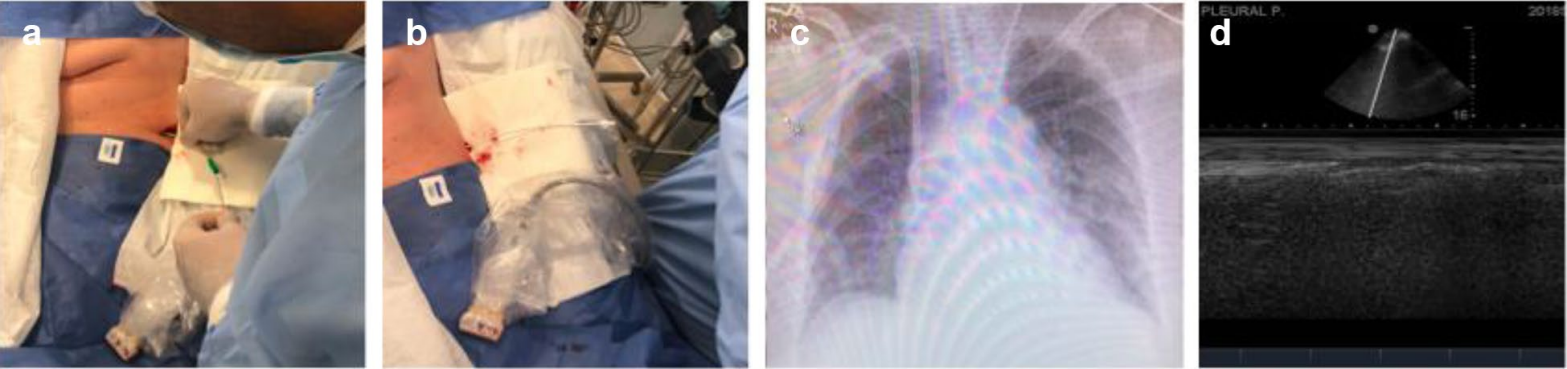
a Passage of the dilator (between 1 and 2 cm), depending on thoracic wall thickness. b Passage of the pigtail catheter to begin drainage. c Chest X-ray after drainage showing a fluid-free pleural cavity. d Ultrasound verification, with a sector transducer, of a fluid-free pleural cavity, also dismissing the presence of pneumothorax (“sand-on-the-beach” sign)
